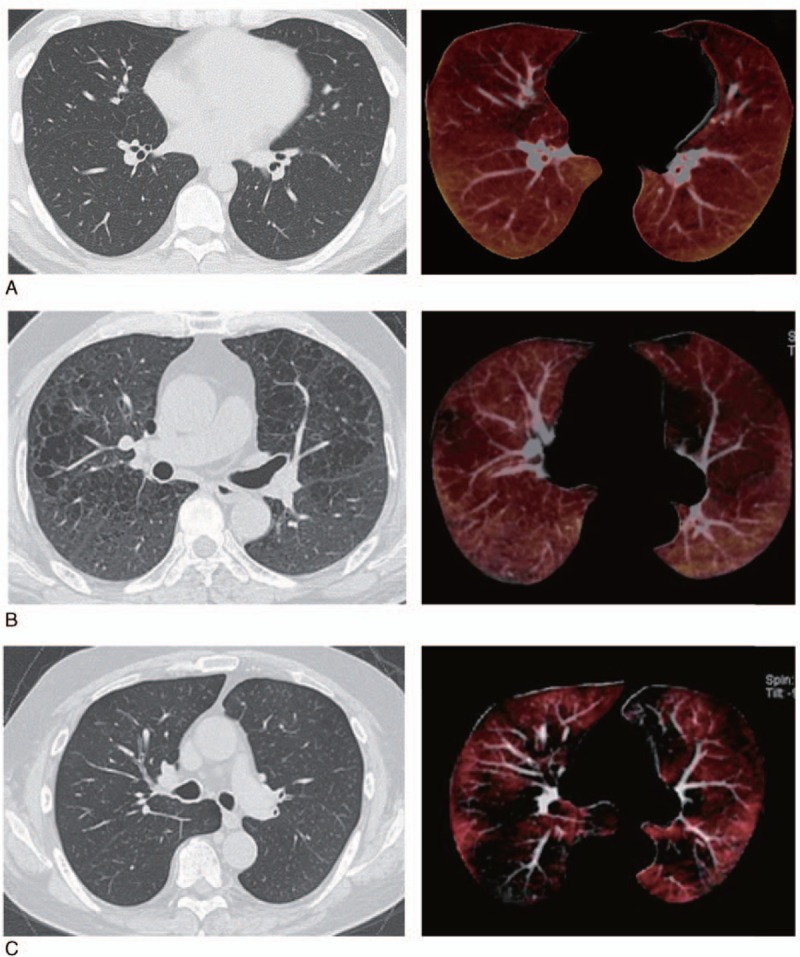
Comparison of conventional or thin-slice CT (left) and xenon images (right). (A) A 33-year-old healthy male, nonsmoker. Conventional CT and pulmonary function test results were within normal limits. (B) A 69-year-old male with COPD. The FEV_1_/FVC ratio was 62%. Pulmonary emphysema is depicted as low-attenuation areas on thin-slice CT (left) corresponding to areas of low attenuation on xenon CT (right). (C) A 66-year-old male with COPD. The FEV_1_/FVC was 33%. Xenon images (right) show low-attenuation areas which are not seen on thin-slice CT (left). COPD = chronic obstructive pulmonary disease, CT = computed tomography, FEV_1_/FVC = ratio of forced expiratory volume in 1 second to forced vital capacity.
